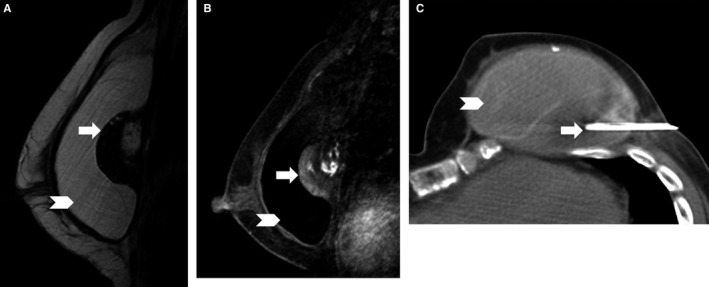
Representative case of benign peri‐implant mass. Left peri‐implant mass that is (A) heterogeneously hypointense on sagittal silicone‐bright image and (B) demonstrates heterogeneous enhancement on the post‐contrast sagittal T1‐weighted fat‐suppressed image. (C) Percutaneous computed tomography (CT)‐guided biopsy was performed and pathology was fat necrosis and necrotic fibrous tissue
